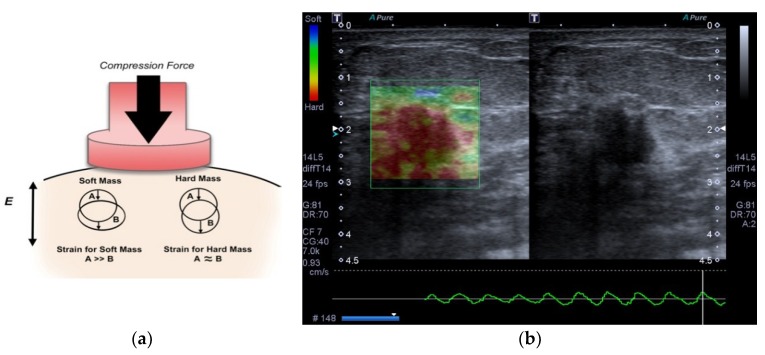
Strain elastography measures tissue displacement as a consequence of an applied initial compression. (a) Behavior of the soft and hard tissue after a compressive force. The displacement of the first is larger in soft tissue than hard tissue. (b) Image of invasive ductal carcinoma in a 56-year-old woman with strain elastography on left and B-mode ultrasound on right.
